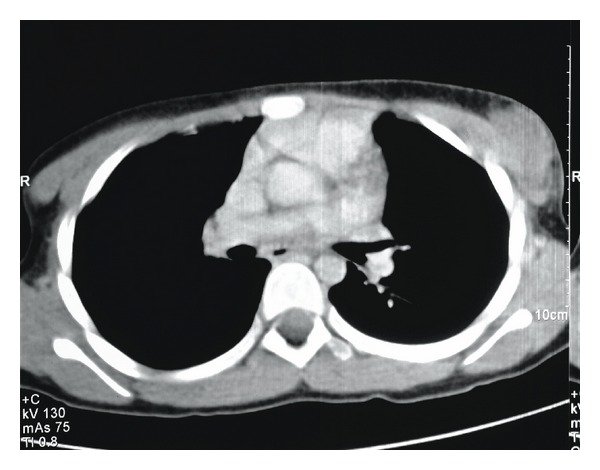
CT of the chest showing several soft tissue lesions noted laterally and anterior to the left pectoralis major measuring 4 cm × 3 cm × 1 cm.
